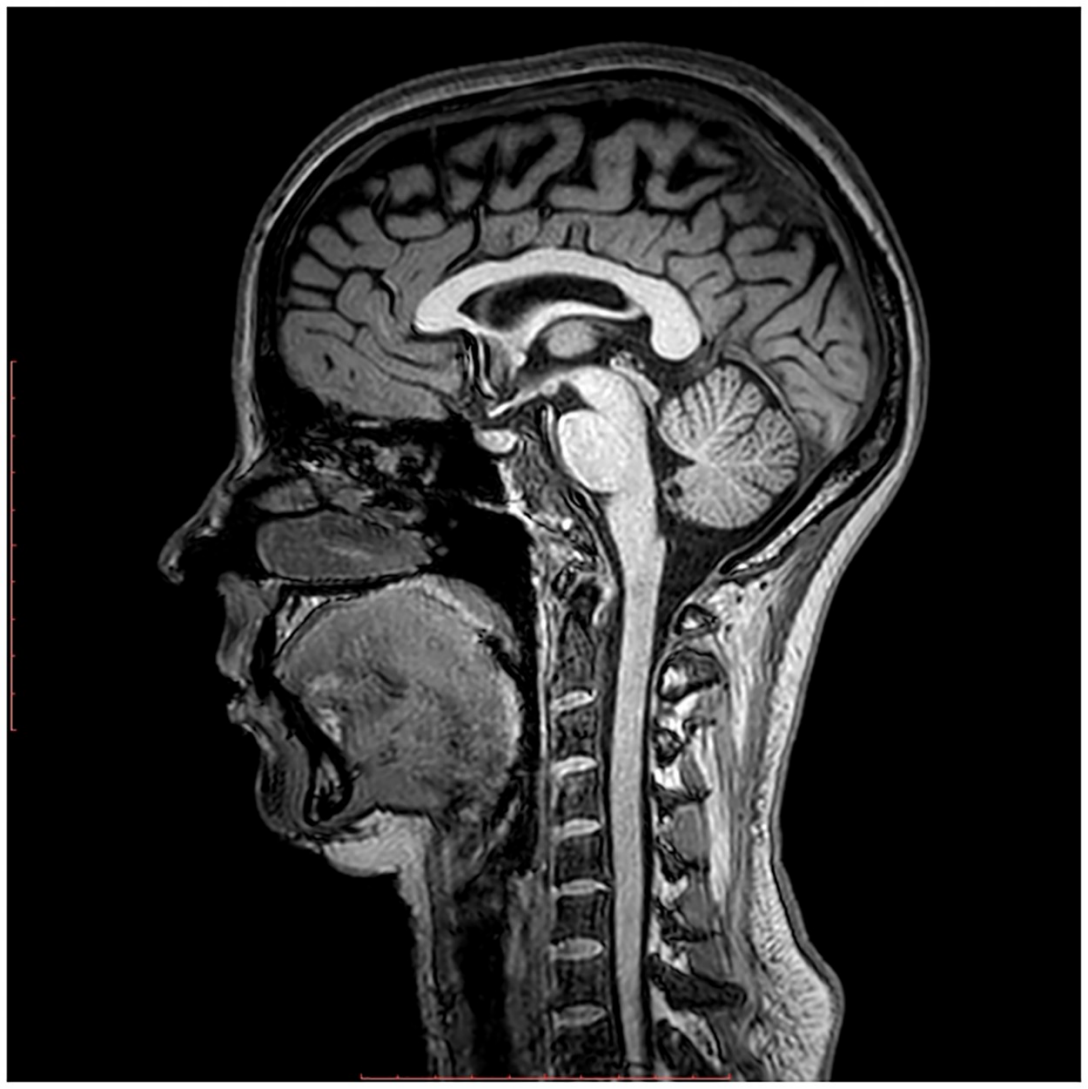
MRI data. Brain MRI saggital scan from one of the volunteer.
